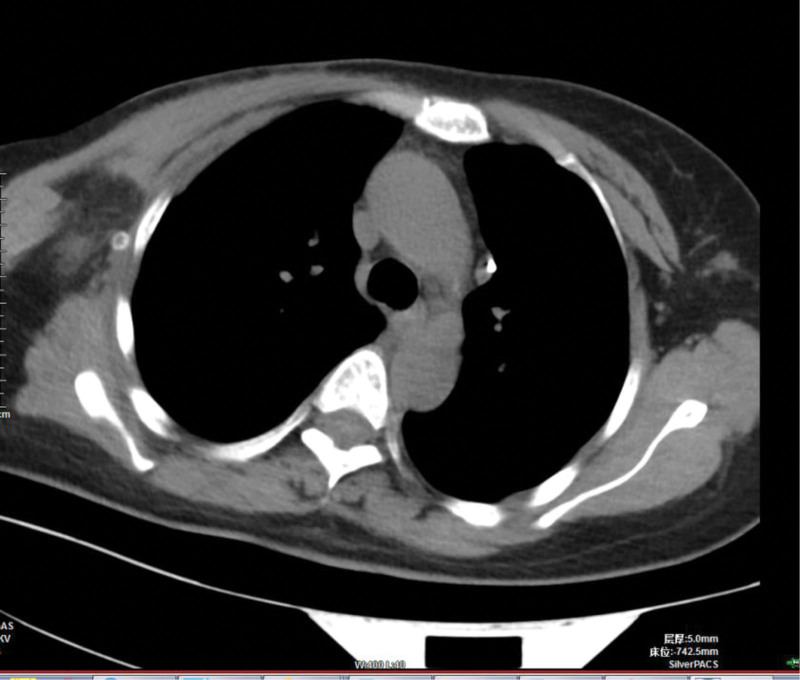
CT: persistent left superior vena cava. CT = computed tomography.
